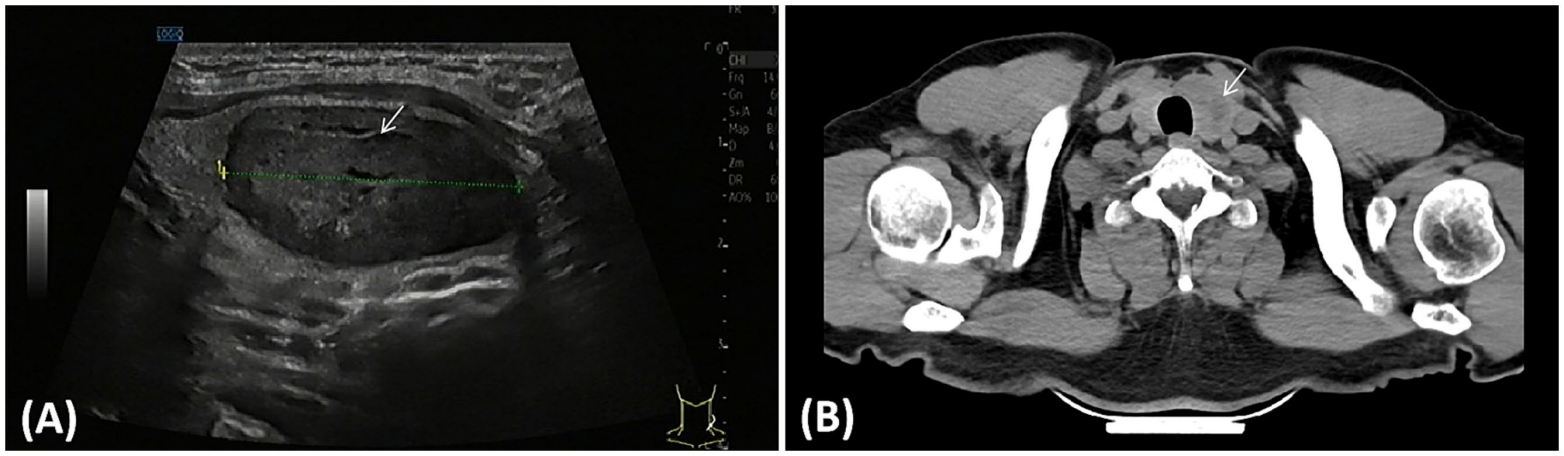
Imaging findings of the hyalinizing trabecular tumor. (A) Ultrasound demonstrated a solitary cystic-solid nodule in the middle and lower part of the left lobe, mainly solid and hypoechoic (arrow). (B) CT reveals an enlarged left lobe with a low-density nodule. The nodule is irregular in shape, has an ill-defined boundary, and compresses the trachea, causing its deviation to the right (arrow).
